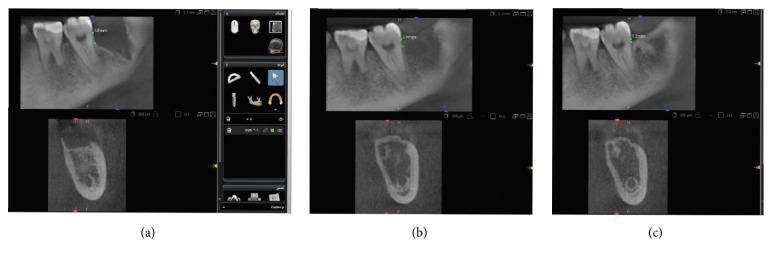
(a) Immediate, (b) 3-month, and (c) 6-month CBCT showing bone height measurement along the distal aspect of the second molar (test site).
